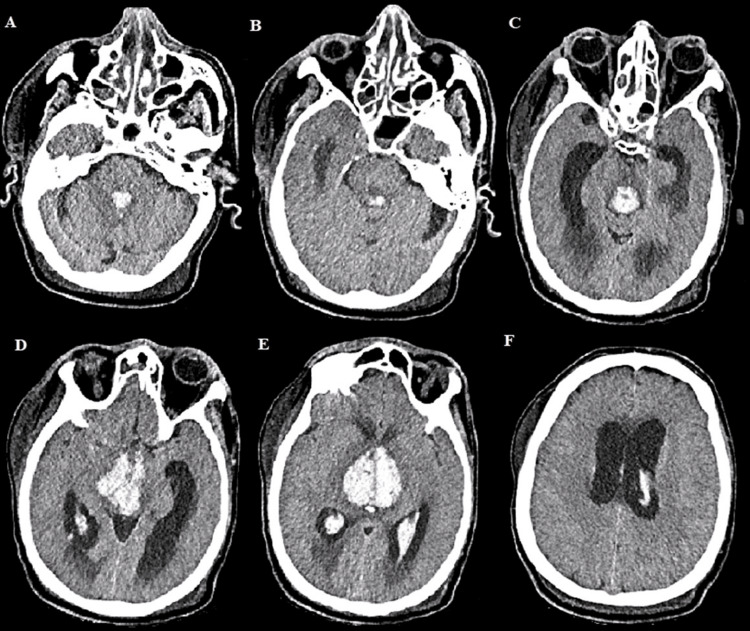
(A-F) Cerebral CT scan showed a bithalamic, periaqueductal and intraventricular hemorrhage, with acute hydrocephalus
